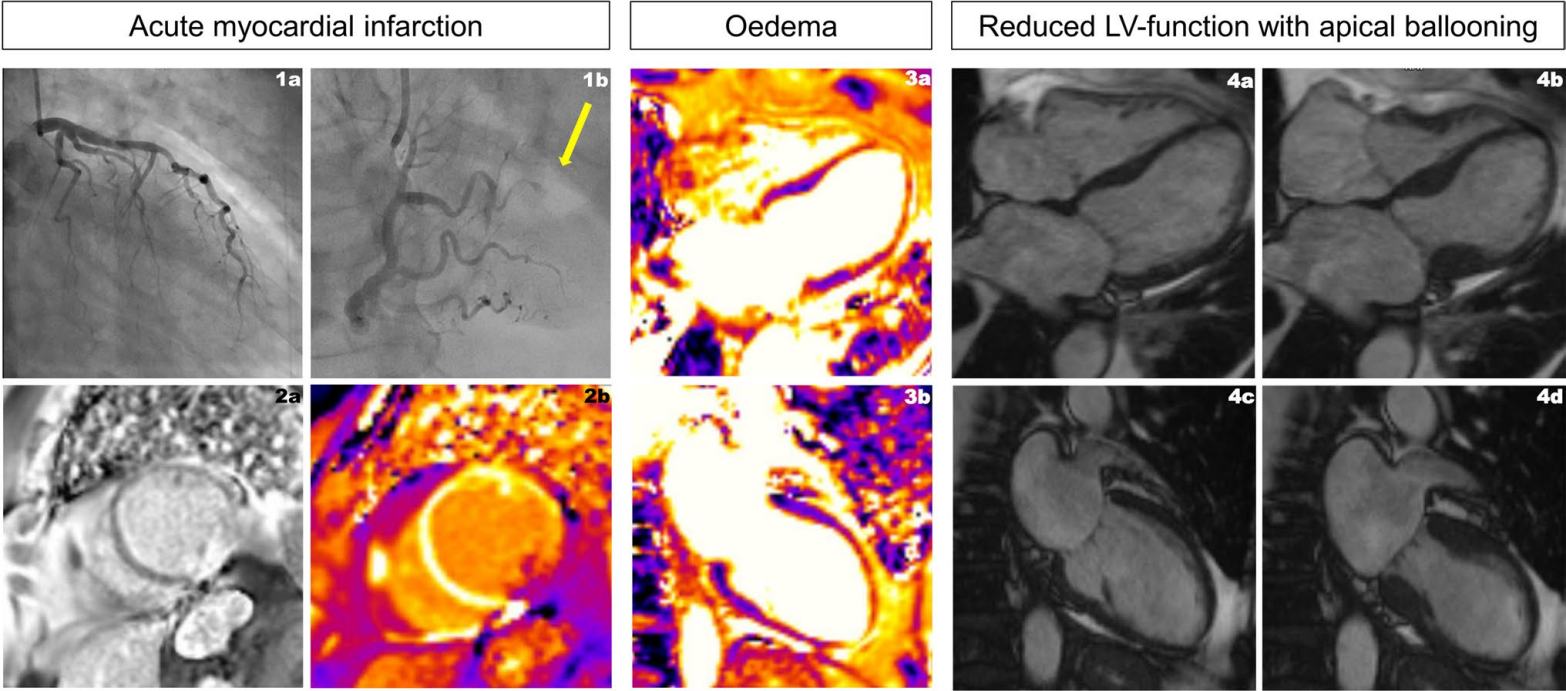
1a – RAO caudal view of the left anterior desending and circumflex artery; 1b – RAO cranial view of the right coronary artery with occluded distal right posterolateral branch (yellow arrow); 2a/b – Cardiovascular Magnetic Resonance (CMR) imaging with evidence of a focal acute transmural inferolateral midventricular myocardial infarction (2a: Late Gadolinium Enhancement; 2b: T1 post-contrast Map); 3a and 3b – CMR T2 map showing myocardial oedema of the apical two thirds of the myocardium; 4a–d –four chamber view cine images in diastole (4a) and apical ballooning in systole (4b); two chamber view cine images in diastole (4c) with apical ballooning in systole (4d)
